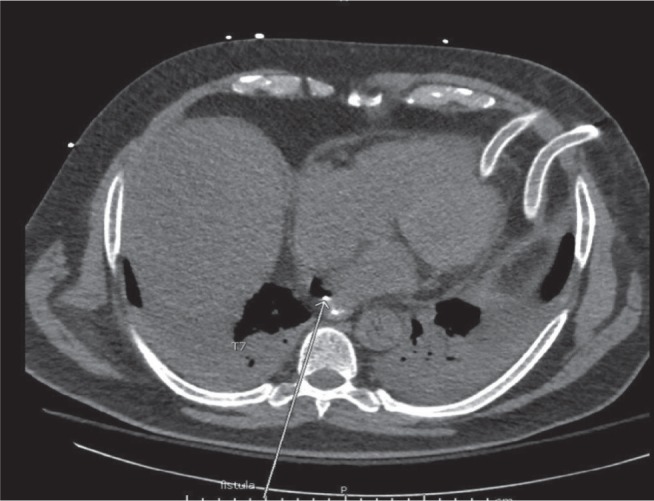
Chest CT scan with oral and intravenous contrast. The arrow indicates the EPF just inferior to the right inferior PV, with extravasation of air and oral contrast into the pericardial space. The left pericardial and pleural drainage tubes are also visible.
